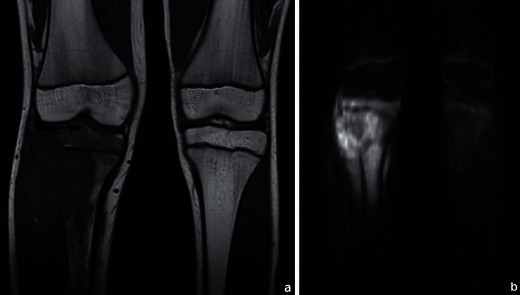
Osteosarcoma of the tibia: coronal T1WI (a) shows a large hypointense lesion in the proximal tibia, which breaks through the bony cortex and invades the adjacent soft tissue. Corresponding DWI (b = 700 s/mm^2^) (b) reveals restricted proton diffusion, consistent with malignancy. Tumoral necrosis (in the centre of the tumour) displays a less hyperintense signal. (Images published by Markus U., Reichardt W. and Kontny U. in MRI: New Developments in Bone Tumor Imaging. Magnetom Flash 2/2011© used with permission of Prof. U. Markus)
